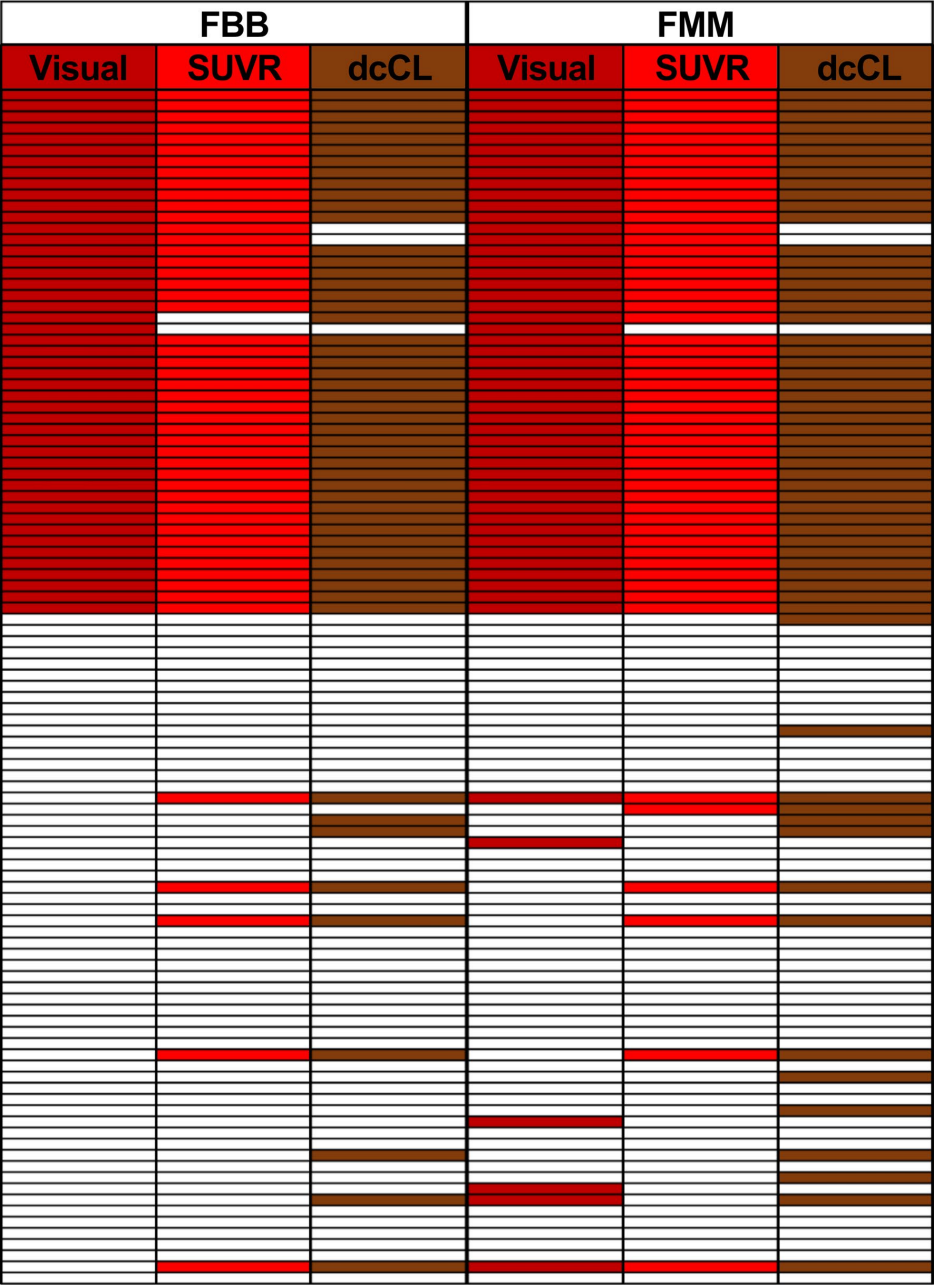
Positivity agreement among visual assessment, SUVR, and dcCL positivity in FBB and FMM. The visual, SUVR, and dcCL positivity results of FBB and FMM are shown as positive in red and negative in grey. Abbreviations: FBB, ^18^F-florbetaben, FMM, ^18^F-flutemetamol; SUVR, standardized uptake value ratio; dcCL, the Centiloid (CL) units using the direct comparison of FBB-FMM CL method.
